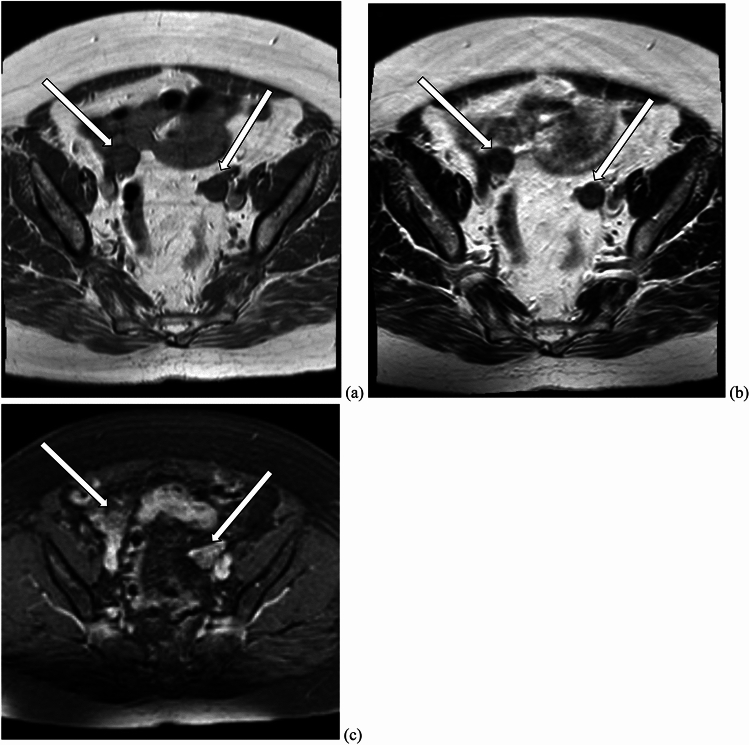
A 69-year-old woman with a history of hypertension, diabetes mellitus, and hepatitis C, who presented with 2 years of hirsutism, with laboratory results demonstrating elevated total testosterone levels and low DHEAS level. MRI abdomen demonstrated stable mild thickening of the left adrenal gland and normal right adrenal gland. Subsequent pelvic MRI was significant for enlargement of both ovaries (arrows) with low to intermediate signal on T1-weighted sequences (image a). On T2-weighted images, the ovaries demonstrated relatively decreased signal intensity (image b). Contrast-enhanced images (image c) demonstrated mild enhancement of the ovaries. The decision was made for the patient to undergo bilateral salpingo-oophorectomy. Final pathology was consistent with ovarian stromal hyperplasia and stromal hyperthecosis
